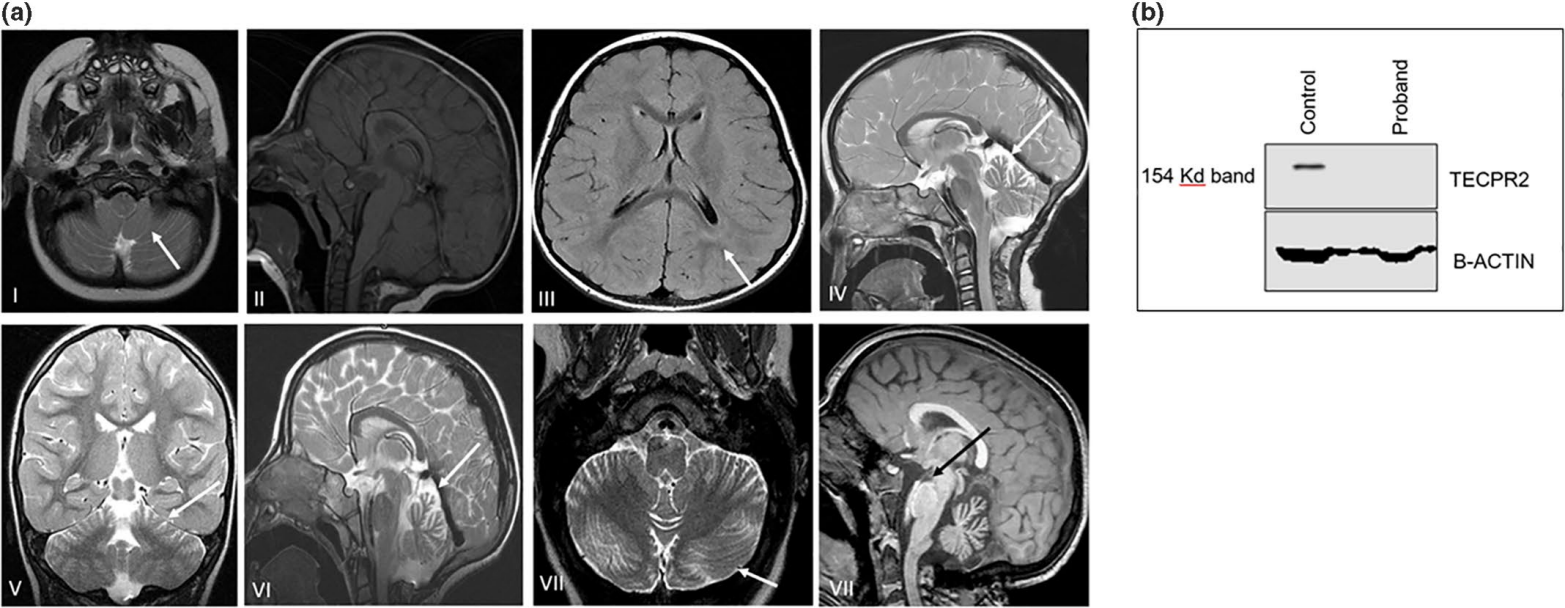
(a) MRI brain images (I) and (II) Chiari malformation type I at 14 months. (II) Normal CV at 14 months. (III) Delayed myelination at 3 years. (IV) Superior CV atrophy at 3 years. (V) and (VI) Progressive cerebellar atrophy (vermis and hemispheres) at 4 years. (VII) Worsening of cerebellar atrophy at 5 years 9 months. (VIII) Flattened pons at 5 years 9 months. (b) Western blot analysis of TECPR2 protein expression from skeletal muscle lysates. No observable band corresponding to the 154 kDa TECPR2 protein was defctected in the patient sample. CV, cerebellar vermis
